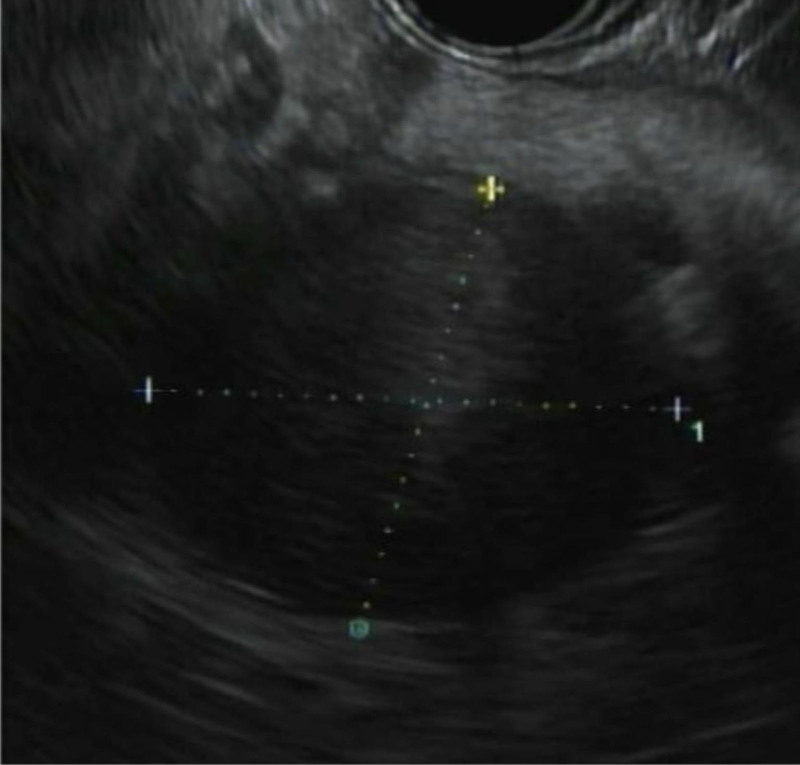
Endoscopic ultrasound of 28 × 40 mm hypoechoic pancreatic tail mass.
